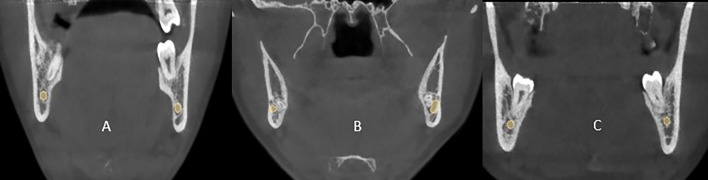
(A) CBCT image of a mandibular canal passing through the lingual side of third molar. (B) The mandibular canal passes through buccal side of third molar. (C) Canal passing through inferior side of third molar.
